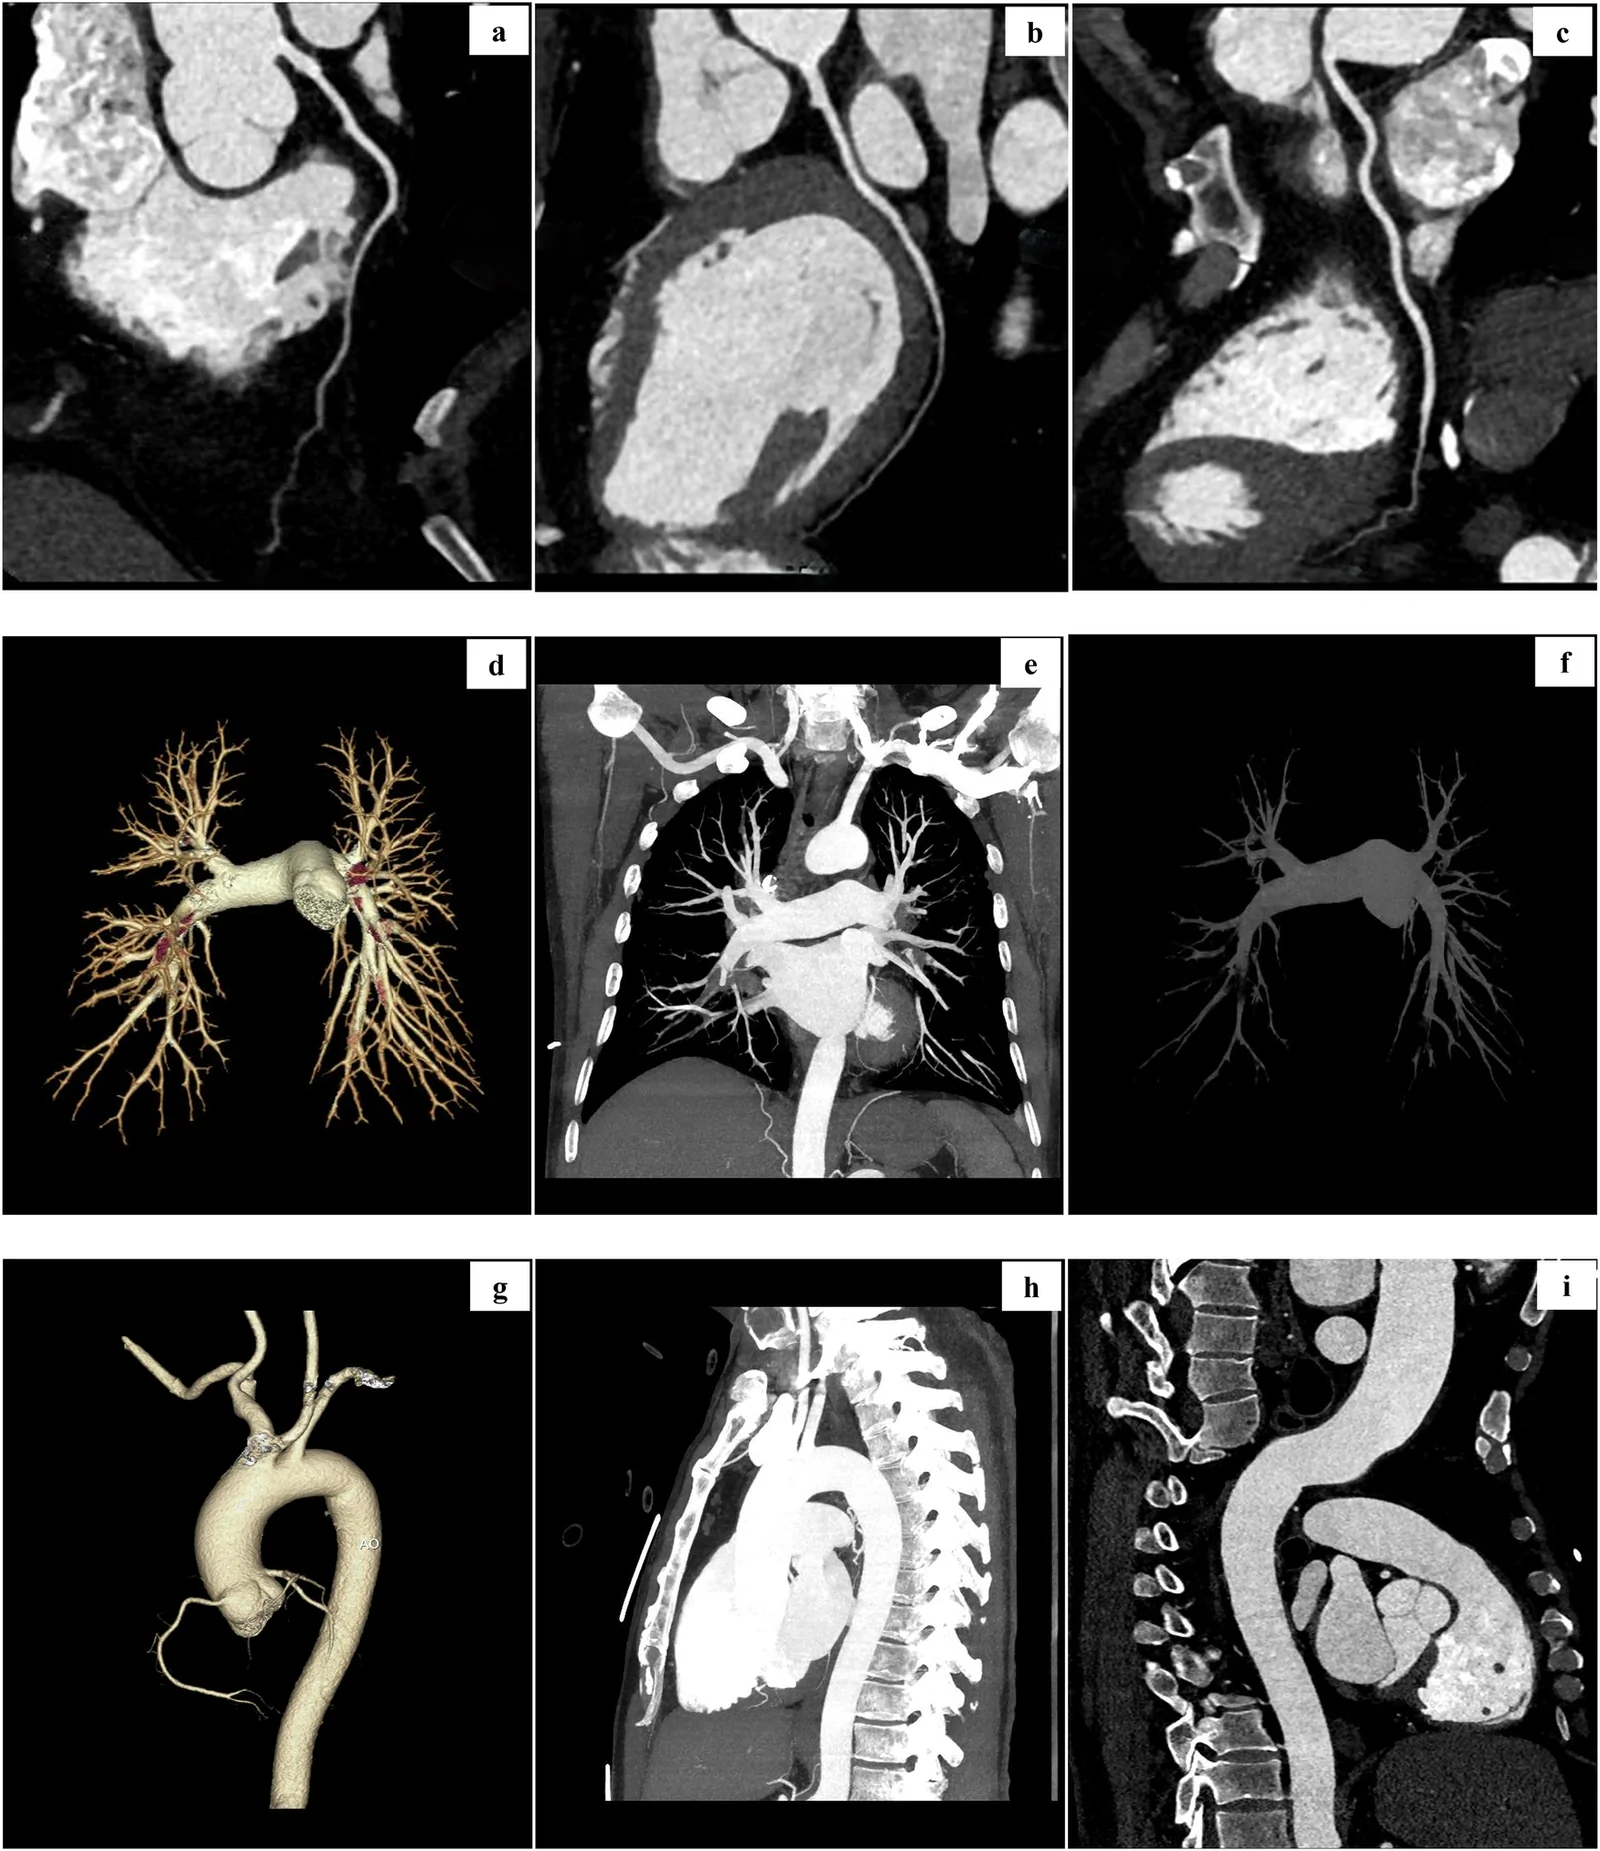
A patient who presented with acute chest pain and dyspnea underwent TRO-CTA using a high-pitch protocol on a third-generation dual-source CT system. a–c Curved planar reconstruction of coronary arteries showing no plaques or stenosis in the left anterior descending artery (a), the left circumflex artery (b), and the right CA (c). d–f PE in the segmental and subsegmental arteries of both lungs. Three-dimensional volume rendering (d) with emboli shown in purple, coronal maximum intensity projection (e), and pulmonary arterial maximum intensity projection (f). g–i No motion artifacts or indications of dissection in the ascending or descending TA
